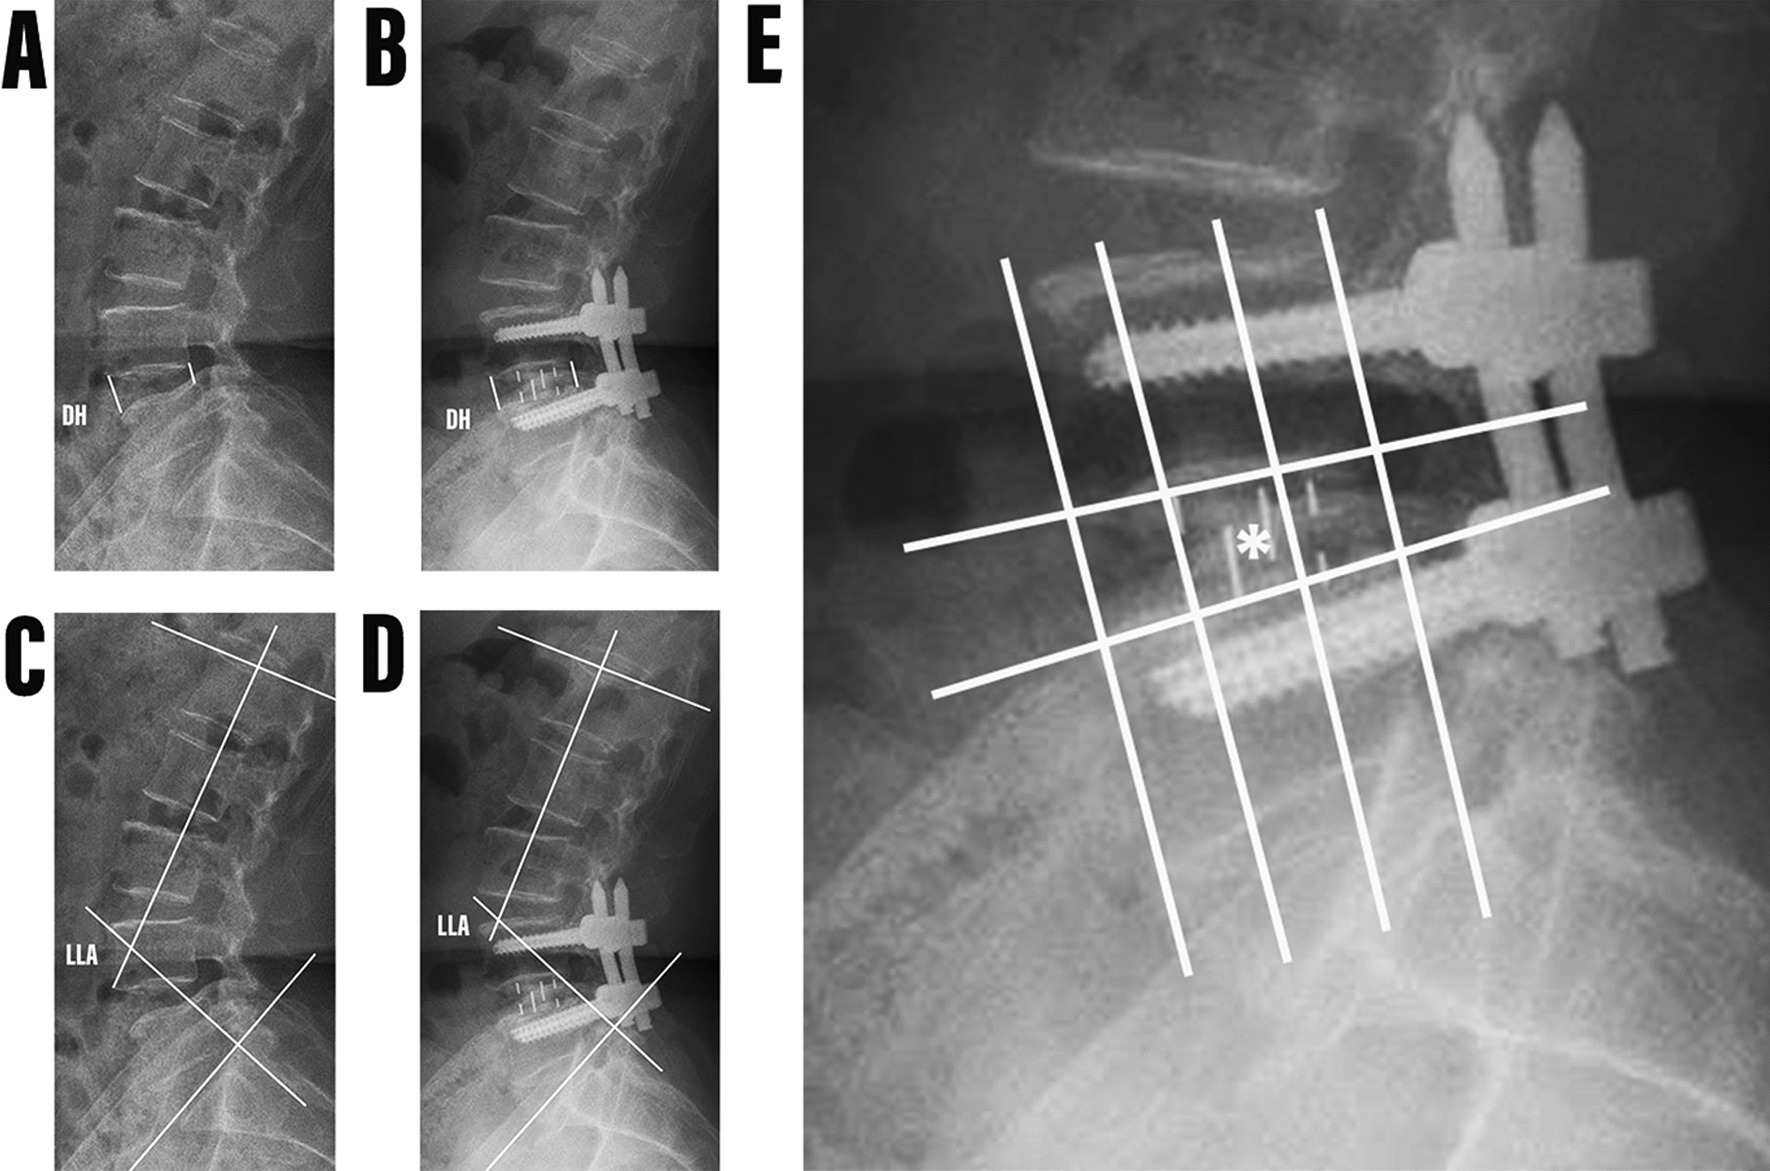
Measurement of Disc height (DH) and lumbar lordosis angle (LLA) by lateral X-ray before and after operation. A Preoperative DH. B Postoperative DH. C preoperative LLA. D Postoperative LLA. E According to the position of the midpoint of the cage (*) in the intervertebral space, the cage position is considered to have an anterior, middle and posterior position
